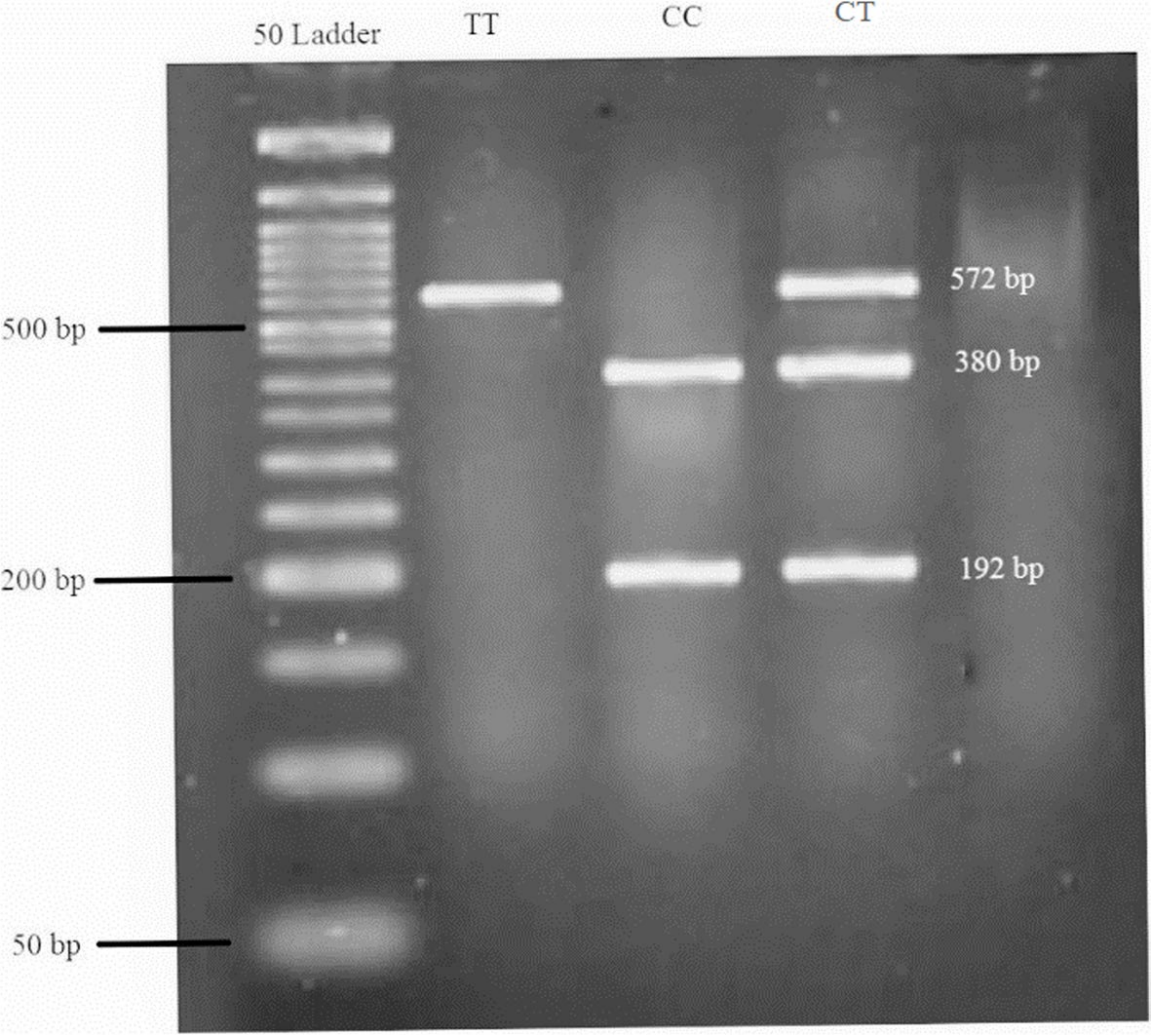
PCR-RFLP analysis of genotyping for the FADS2 rs174583 variants by TauI. Length of the digested products were determined by 50-bp ladder. TT = homozygous mutated (572 bp), CC = homozygous wild-type (380 and 192 bp), CT = heterozygous mutated (572, 380, and 192 bp)
